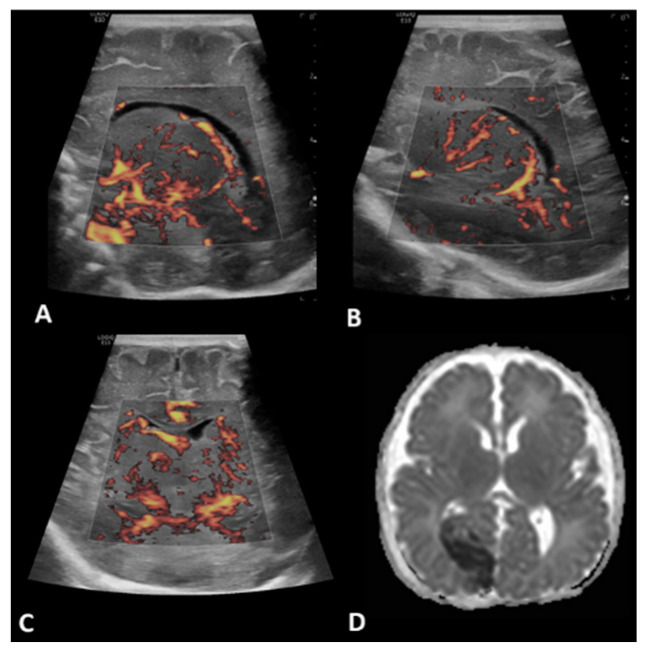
A 2-day-old boy with no history of congenital cardiac disease underwent ECMO due to severe pulmonary hypertension and respiratory failure. Brain MVI was performed after six days from cannulation; grayscale US was normal. Brain MVI demonstrated asymmetric and heterogeneous blood flow in the deep gray nuclei as seen in left (A) and right (B) parasagittal views, as well as in the coronal view (C). The patient remained on ECMO for a total of seven days. MRI obtained two days after ECMO decannulation showed diffusion restriction in the right medial occipital lobe consistent with acute stroke in the expected distribution of branches of the right posterior cerebral artery (D).
